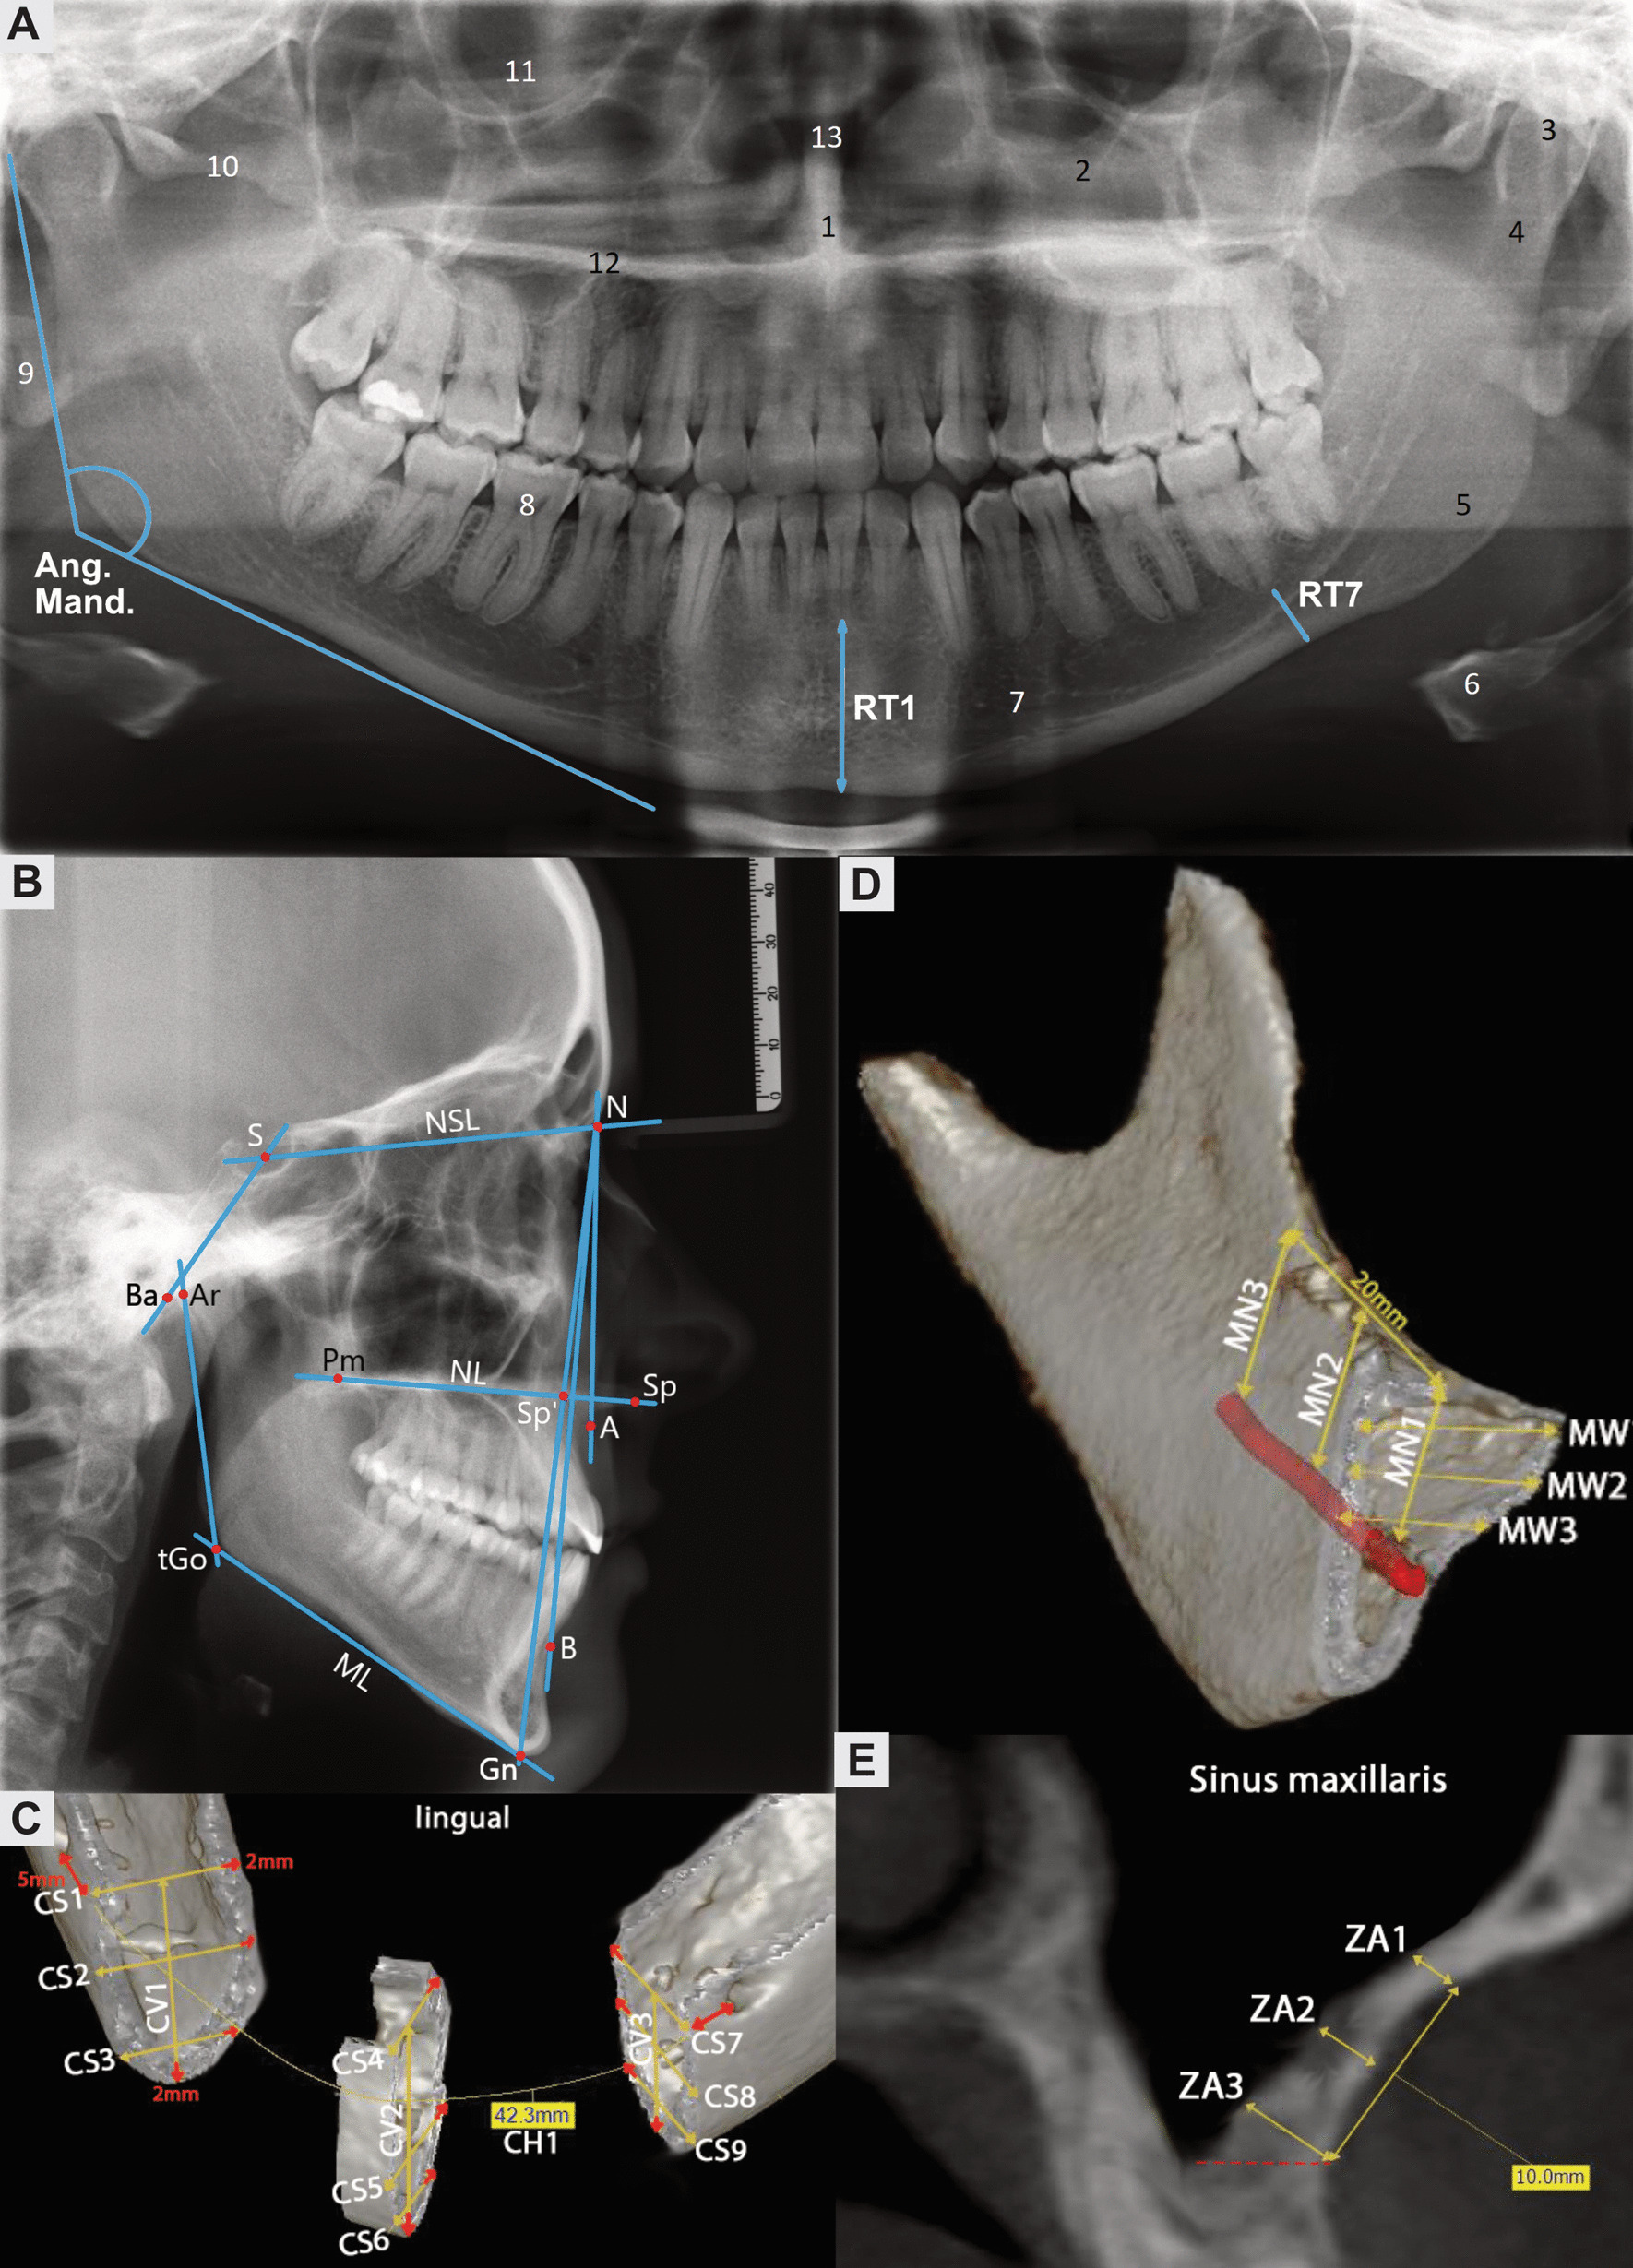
A PR with acquired measurements. Distance of the root tip of the central incisor (RT1) and second molar (RT7) to the mandibular base, jaw angle (Ang. Mand.), spina nasalis anterior (1), sinus maxillaris (2), caput mandibulae (3), collum mandibulae (4), mandible (5), os hyoideum (6), foramen mentale (7), bony auditory meatus (9), arcus zygomaticus (10), orbita (11), palatum durum (12), septum nasi (13). B LCR with cephalometric parameters. SNA angle, SNB angle, ANB angle, ML-NSL angle, NL-NSL angle, ML-NL angle, NSBa angle, Gn-Go-Ar angle, index = $$\frac{{N - Sp'}}{{Sp' - Gn}} \times 100$$. C Three-dimensional CBCT reconstruction of the chin region showing all measured distances (yellow) and safety margins (red). D Three-dimensional CBCT reconstruction of the right mandibular retromolar region with inferior alveolar nerve (red) and measured dimensions of the bone block. For visualization purposes, only the measurements of the first of three sections was presented. E Coronal CBCT cross-section of the zygomatic alveolar crest with measurements and floor of the maxillary sinus as caudal border of the bone graft (red dotted line)
